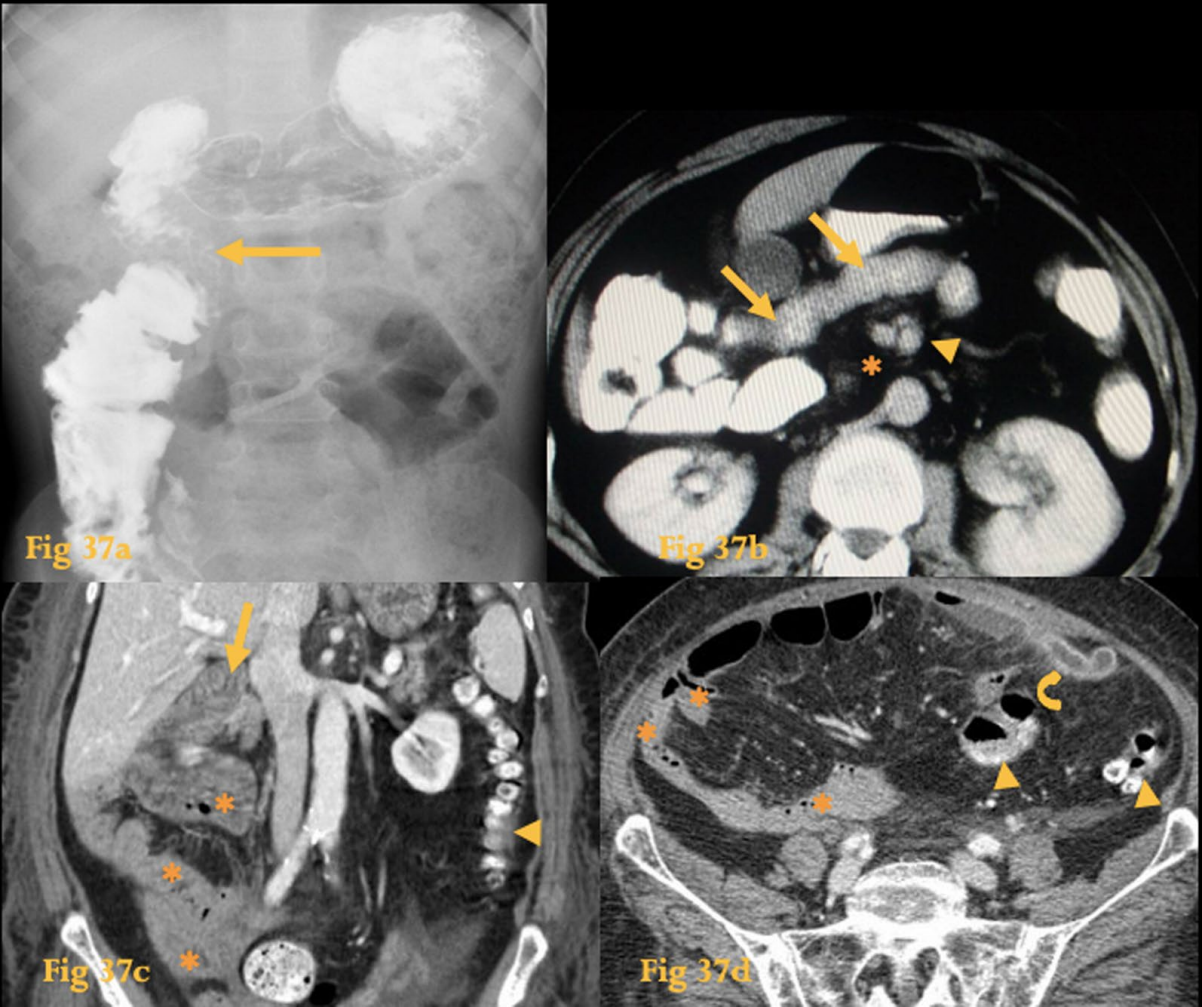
Gut malrotation. Barium study of an 8-year-old (a) with intestinal malrotation shows that the duodenum does not cross the midline (arrow). Abdominal CT of an adult patient (b) reveals absence of the 2nd part of the duodenum between the aorta and SMA (asterisks). The duodenum (arrows) crosses anterior to the SM vessels, which show an inverted relationship, with the artery located on the right and the vein on the left side (arrowhead). Incidentally, this patient also had an abnormal course of the portal vein and absence of the inferior vena cava. Abdominal CT of another adult with left flank pain and fever (c, d) shows that the duodenum does not cross the midline (arrow). The small bowel is located predominantly on the right quadrants (asterisks) while the large bowel is located on the left quadrants (arrowheads). On the left flank, an enhancing and thickened, fluid-filled tubular structure (curved arrow) is seen coming out of the cecal pole, which was located on the midline—this was a rare case of an appendicitis on the left quadrants in a patient with nonrotation
